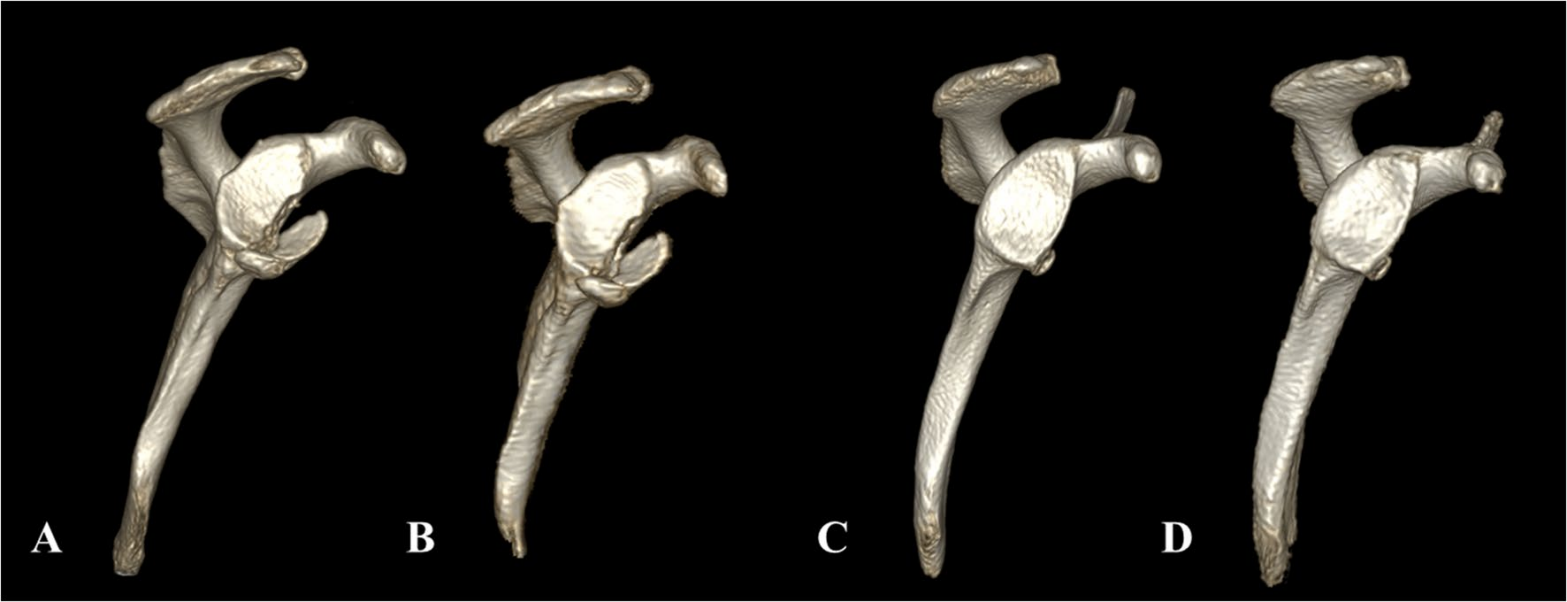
Comparison of glenoid 3D VR from two patients included in this study. (A, B) Large glenoid fracture on CT (A) vs DL ZTE (B). (C, D) Small glenoid defect on CT (C) vs DL ZTE (D)
